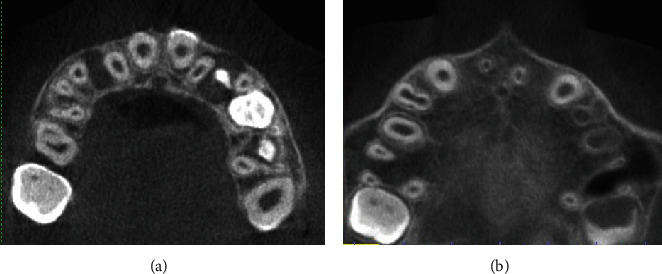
Axial CBCT views showing the position of crowns (a) and roots (b) of #11 in relation to #21 indicating labial intrusion of #21.
